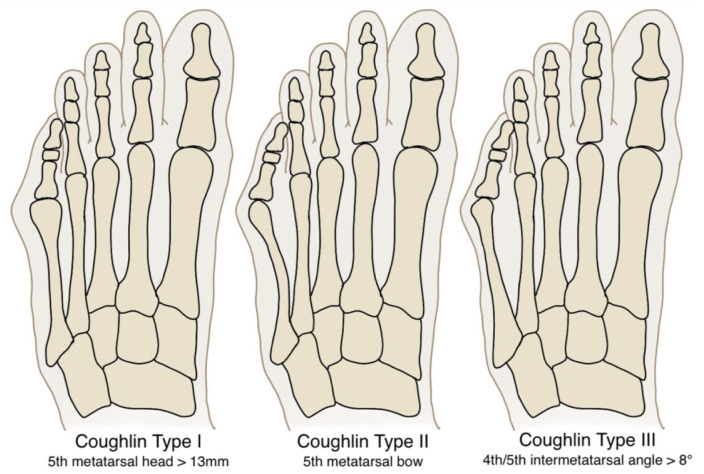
Coughlin’s radiographic classification.
